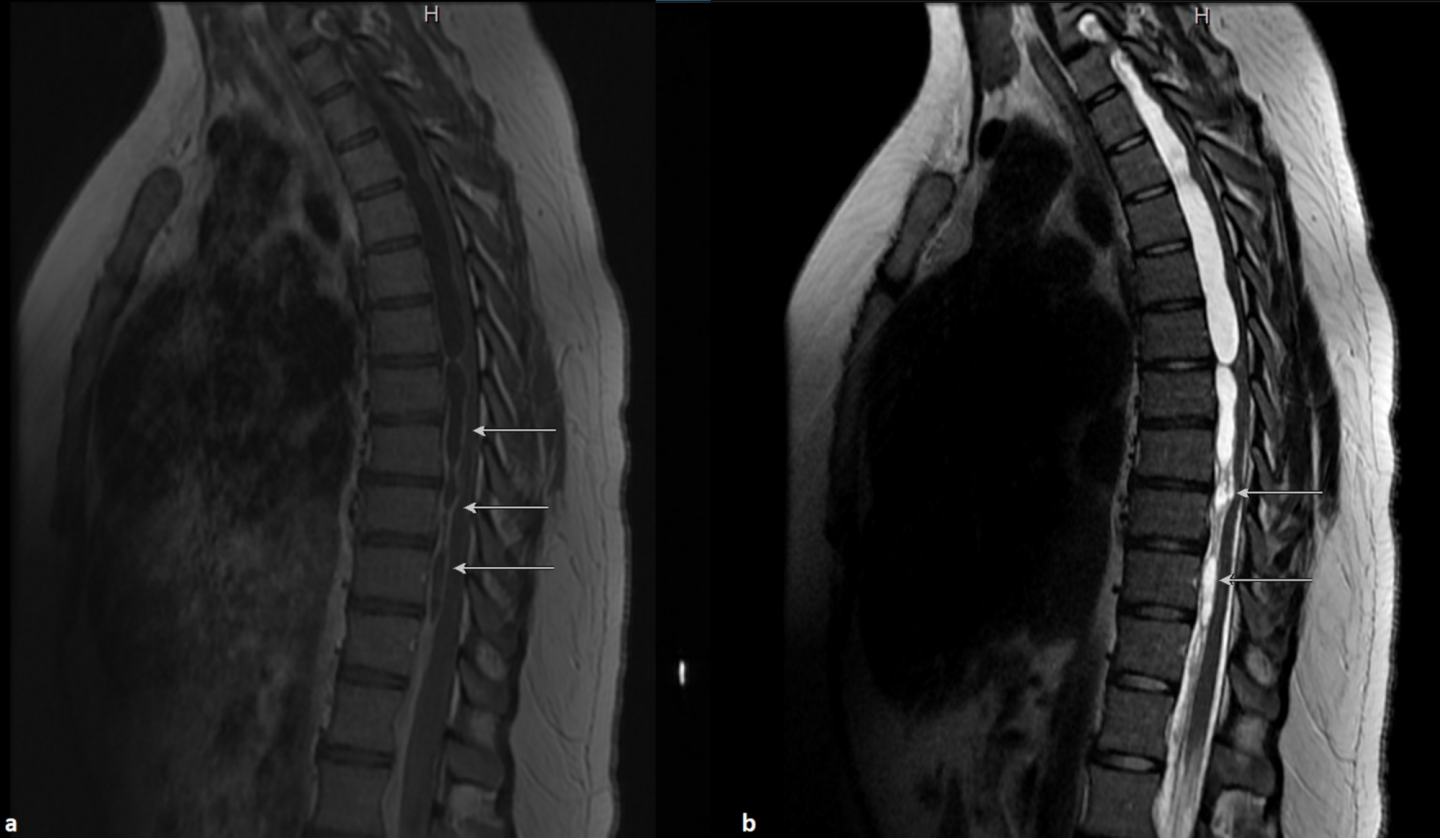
(a) T1 and (b) T2 sagittal thoracic MRI demonstrating cystic arachnoid formations (white arrows) from upper to lower thoracic spine.
